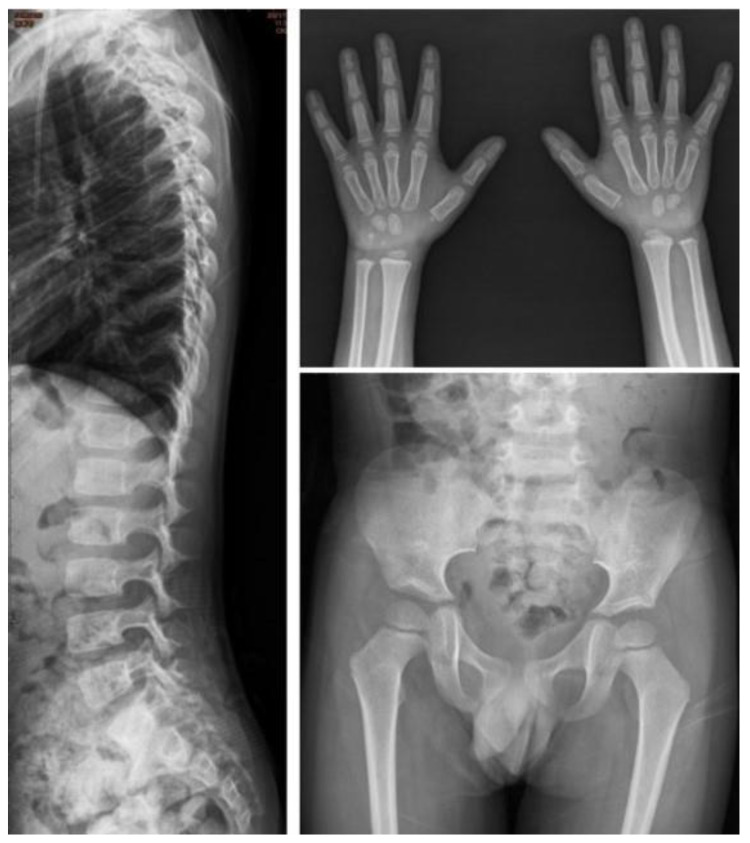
The bone X-rays at 4.3 years of age of patient No. I-2 who had the variation allele c.311A > T [p.D104V]. ERT was started at 1 year of age. He received HSCT at 1.5 years of age. At 4.3 years of age, X-rays of his spine, hands, and pelvis were all normal.
